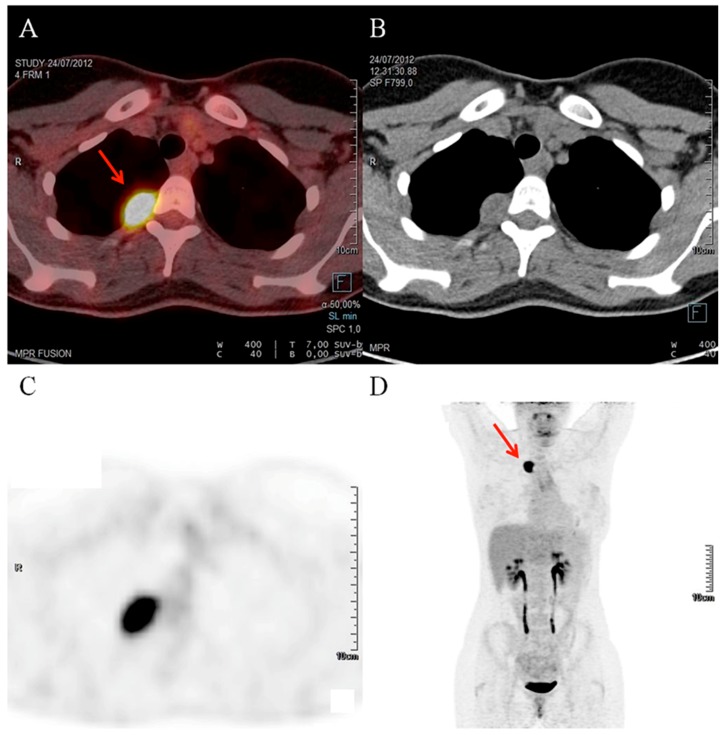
2-deoxy-2-[fluorine-18]fluoro-d-glucose (FDG) positron emission tomography/computed tomography (PET/CT). (A,C,D) Isolated hypermetabolism (standard uptake value up to 18 SUV max) of a 2 cm tumor (arrows), close to the costovertebral angle of the third right thoracic vertebra. Note the absence of other uptake. (A) coronal fused PET/CT images; (B) planar view showed a 2 cm para-vertebral lesion; (C) anterior whole-body maximum intensity projection (MIP) images.
